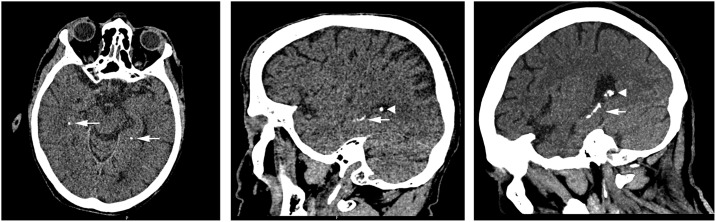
Hippocampal calcification on thin unenhanced CT examinations (arrows). The left axial reconstructed image shows bilateral mild hippocampal calcification (dot). The middle sagittal reconstructed image shows moderate hippocampal calcification (multiple dots). The right sagittal reconstructed image shows severe hippocampal calcification (confluent). Calcification of the choroid plexus is also visible (arrowheads).
